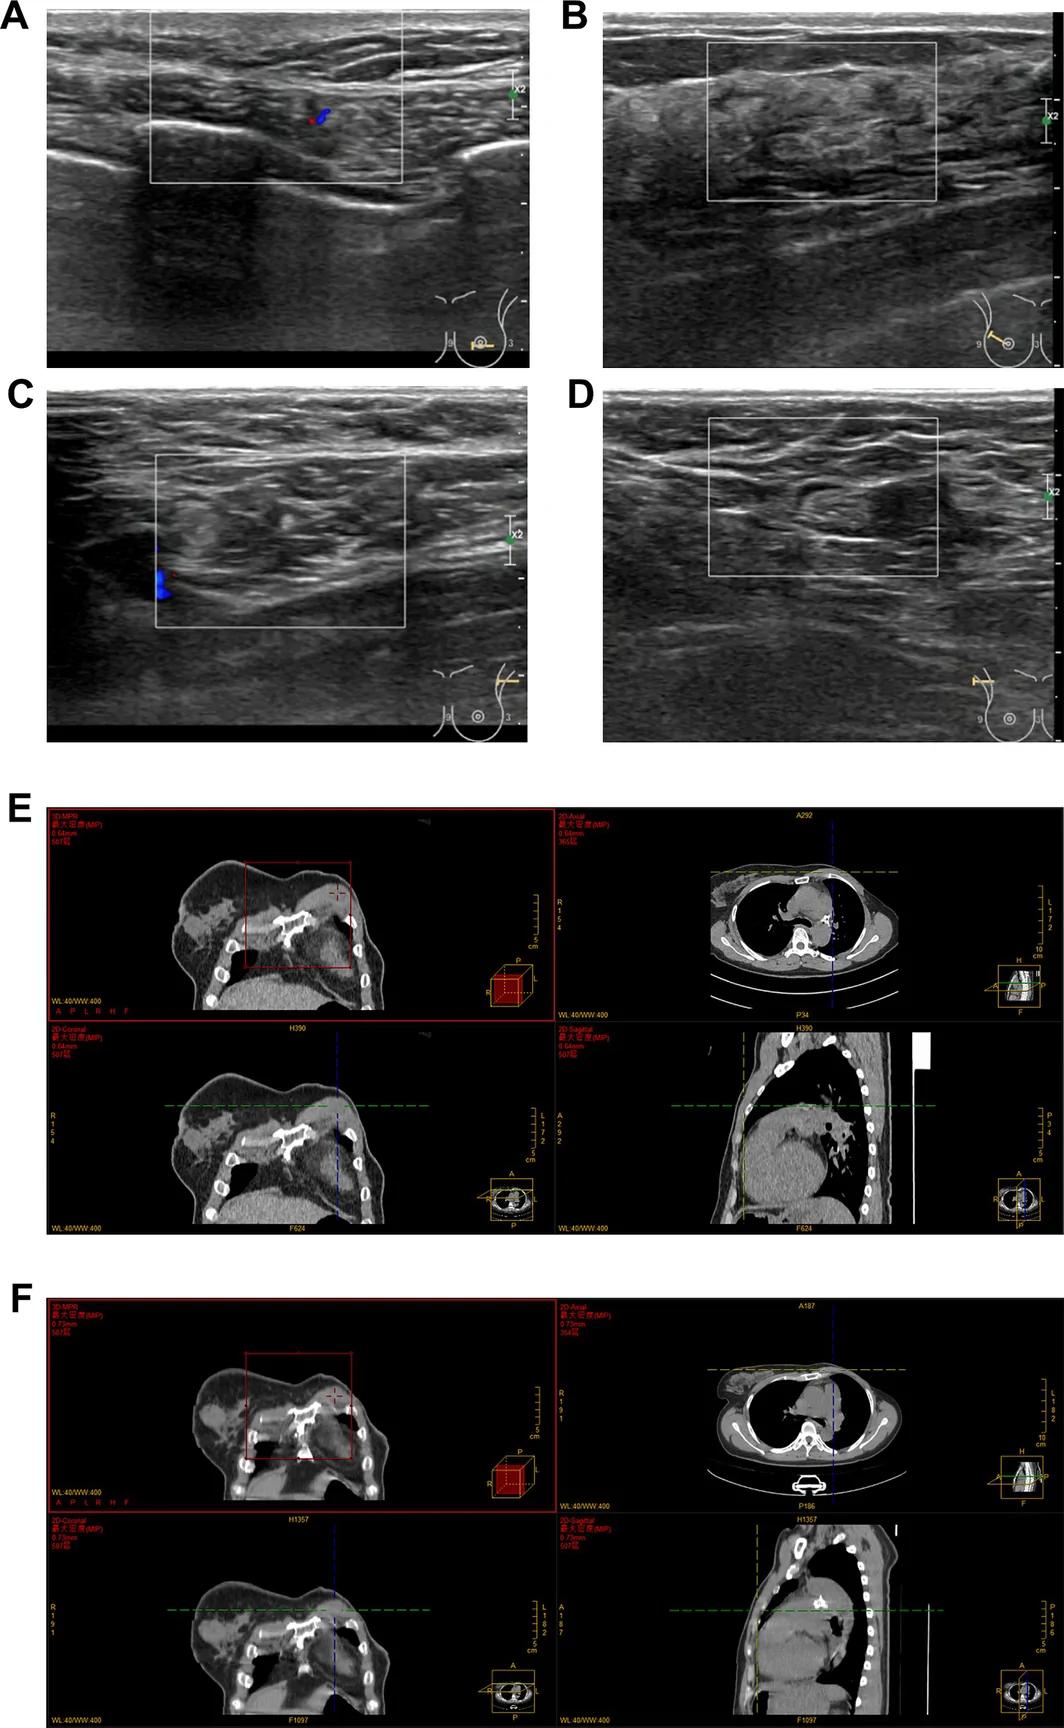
Postoperative follow‐up imaging examinations. (A–E) Follow‐up CDUS and chest CT images acquired on November 19, 2020, including left chest wall CDUS (A), right breast CDUS (B), bilateral axillary CDUS (C–D), and post‐mastectomy chest CT (E). (F) Post‐mastectomy multiplanar localized chest CT obtained on July 23, 2025.
